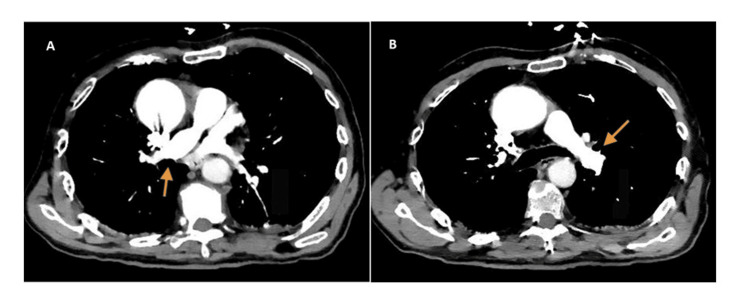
Chest computed tomography (CT) pulmonary angiography. A. The orange arrow indicates the right pulmonary artery branch. B. The orange arrow indicates the left pulmonary artery branch. No evidence of pulmonary thromboembolism is observed.
